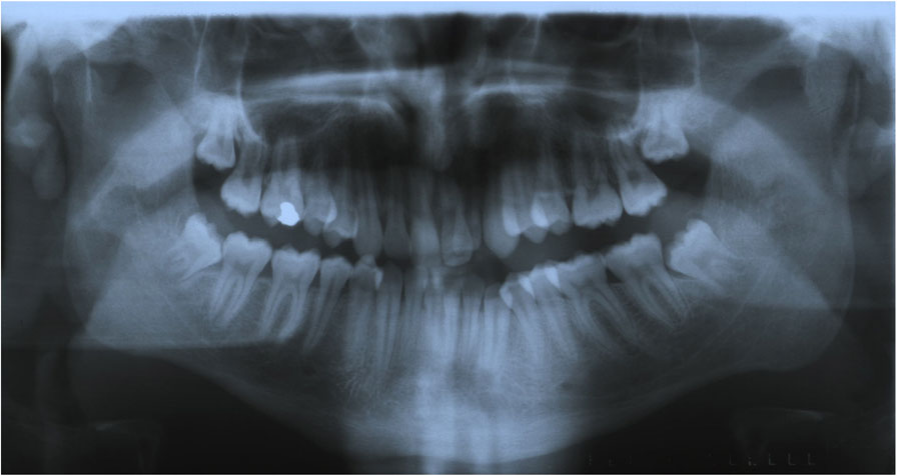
Post-surgical orthopantomograph demonstrating surgically removed coronoid processes
